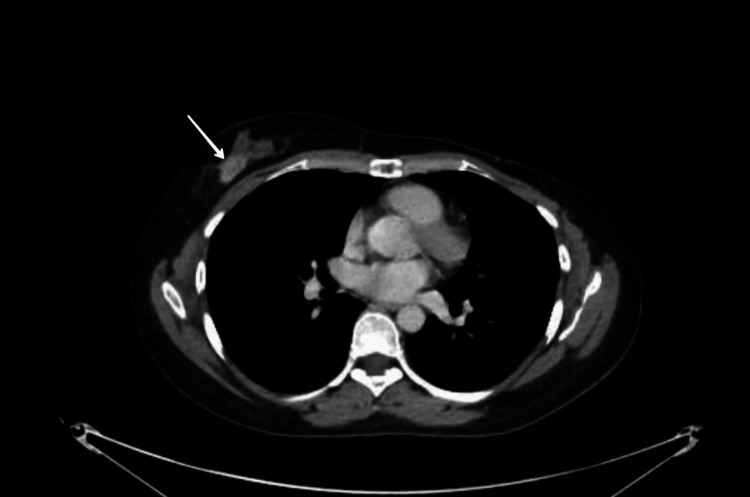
CT of the thorax, abdomen, and pelvis with contrast cross-section showing a new breast malignant lesion. CT: computed tomography
